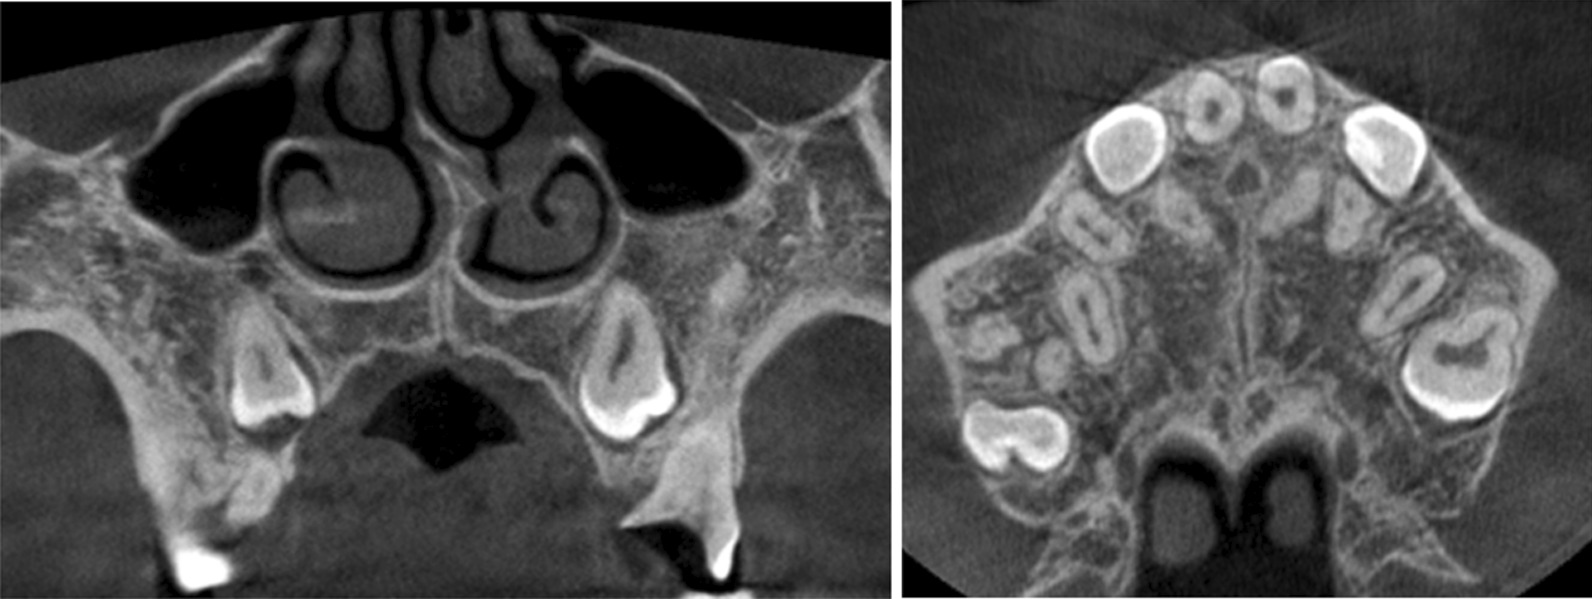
CBCT after MARPE after the first maxillary expansion
